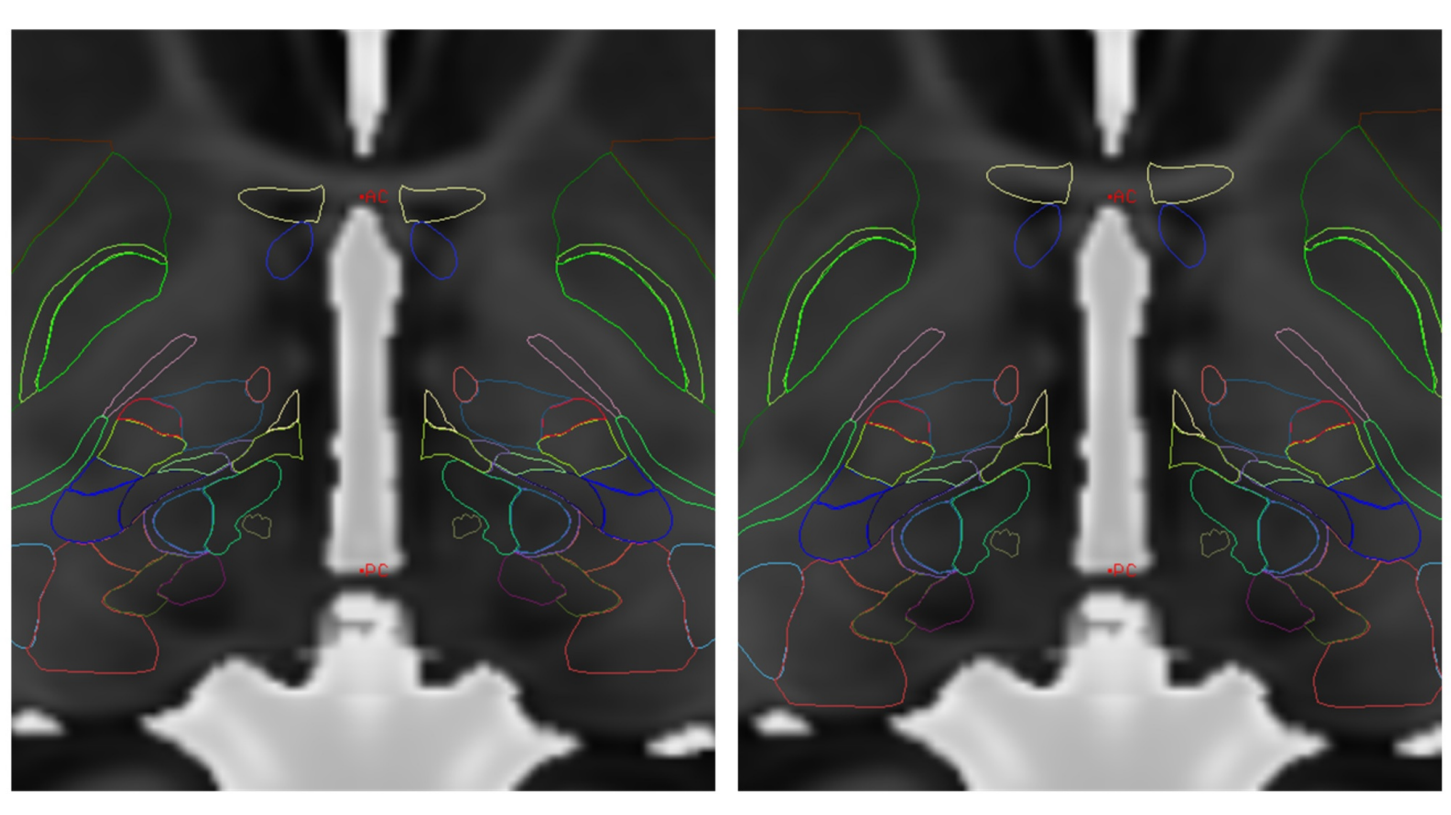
Simple scaling of AP dimension using AC-PC distance in MRI and atlas. The left image has no scaling, but is anchored on MidACPC. The right image has the AP scaling correction on this MNI Atlas over the MRI. This simple method is effective for basal ganglia targeting. MNI = Montreal Neurological Institute; AC = Anterior Commissure; PC = Posterior Commissure; MRI = Magnetic Resonance Imaging; AP = Antero-Posterior; MidACPC = Middle of Anterior Commissure and Posterior Commissure MNI Atlas = Montreal Neurological Institute Atlas, please refer to reference [19]. Figure provided by Mevis Stereotactic Planning System (MNPS)
